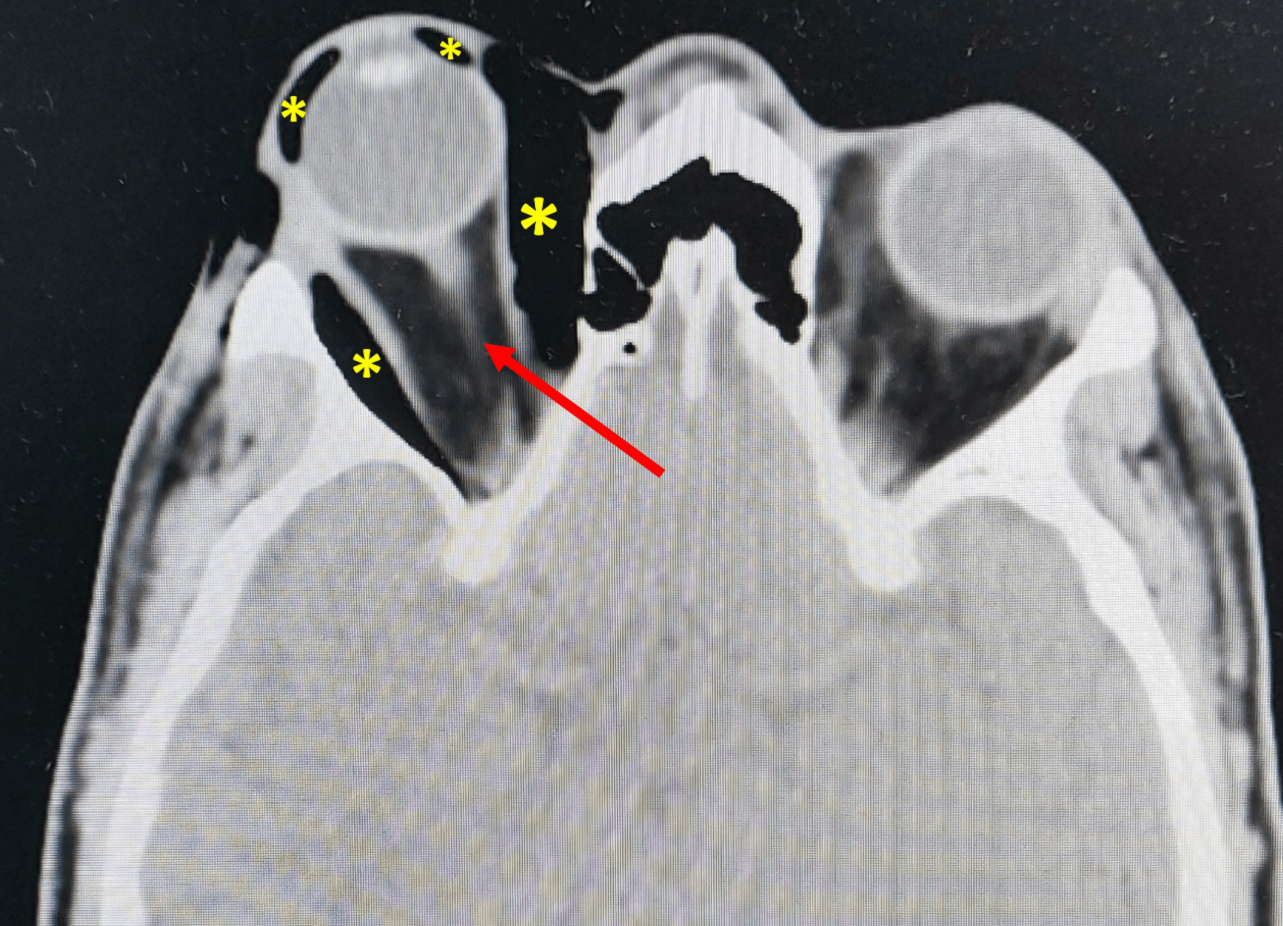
Axial CT orbit showing right globe proptosis with orbital emphysema (yellow asterisks) and stretching of the optic nerve (red arrow) CT: Computed tomography
